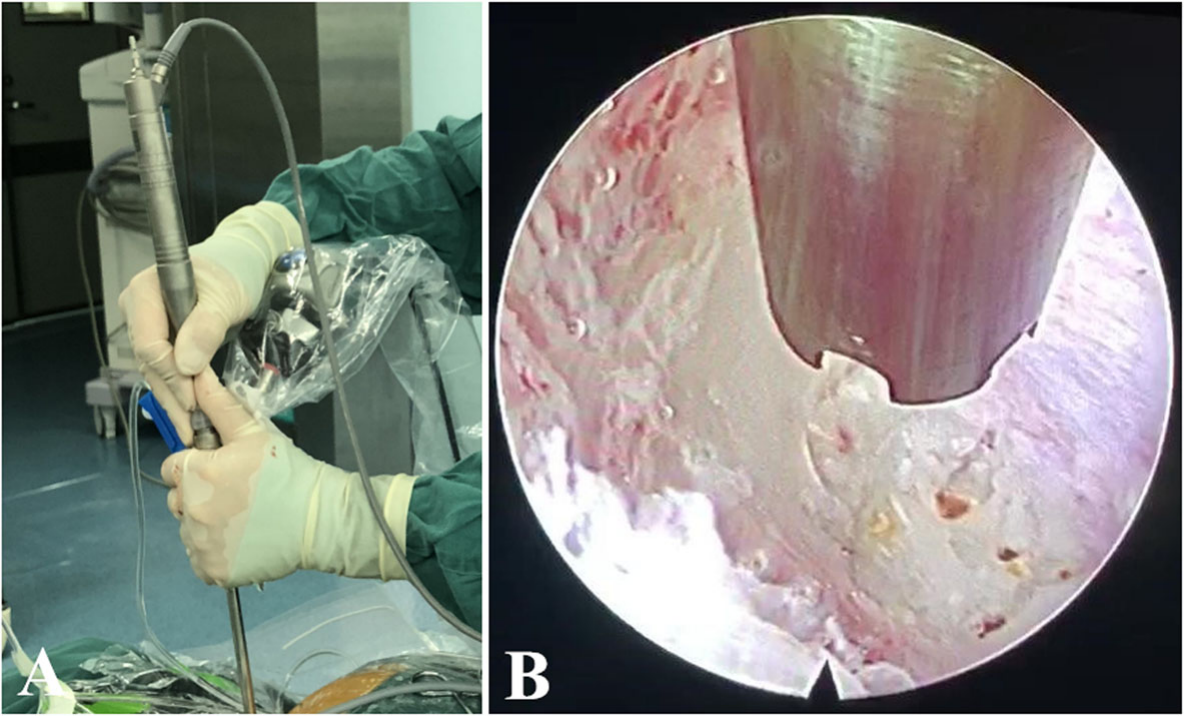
The partial lamina was removed safely and efficaciously with the assistance of the ultrasonic osteotome (a) under the monitoring of endoscope (b)
